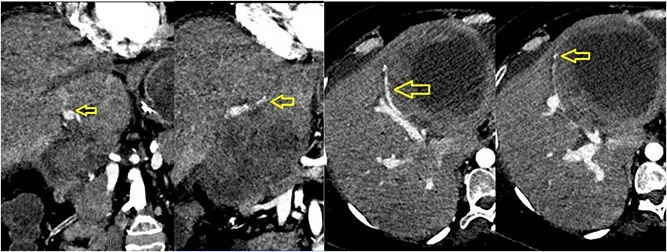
Abdominal CT angiography showing the left hepatic artery supplying the lesion.
